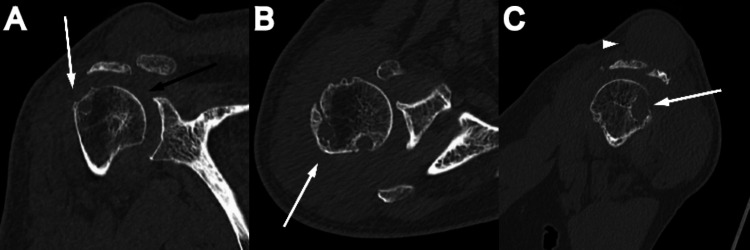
Computed tomography images Moderate degenerative changes in the acromioclavicular joint (ACJ) and glenohumeral joint (black arrow) including a bone cyst around the greater tuberosity (white arrow) (A, B, C) and a cyst projecting superior to the ACJ (arrow head) (C).
